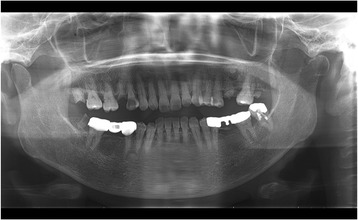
Panoramic dental radiograph. A radiolucent destructive lesion and pathologic fracture were seen in the left condyle
